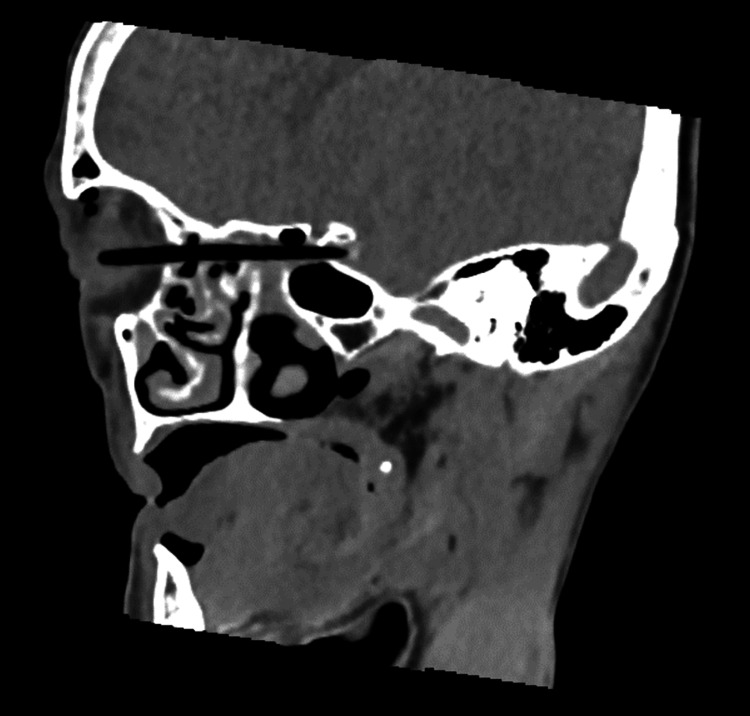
Preoperative sagittal CT findings The sagittal section clearly shows a continuous penetration pattern from the puncture point, rather than air.
